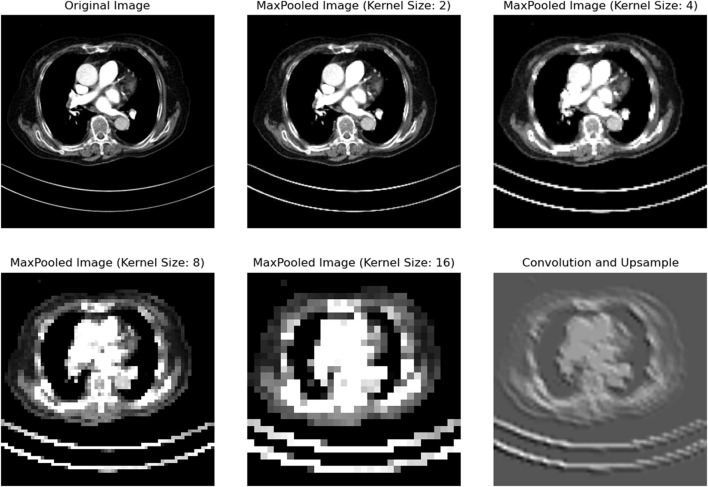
After the CT images are reduced in resolution at different magnifications, we can see that the image is still relatively clear when the resolution is reduced to 1/2 and 1/4 of the original, but after being reduced to 1/8 or smaller, obvious pixel blocks appear, indicating that the details have been lost. The last picture is restored by upsampling the high-dimensional image. It can be seen that the organ boundary and position details have been lost a lot, which is the reason why we try to avoid upsampling.
